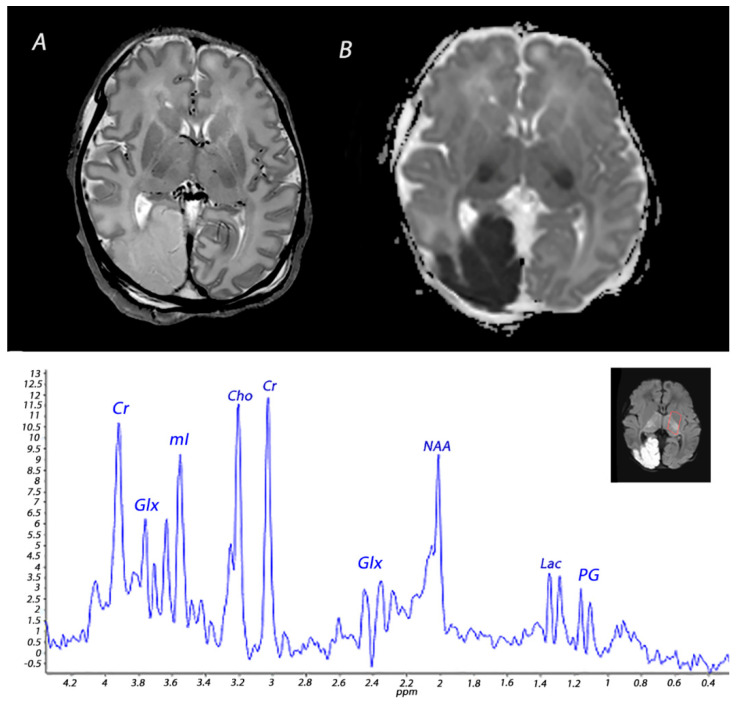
MRI performed on day 3 in an infant born with Apgar scores of 3/4/6 who received therapeutic hypothermia for severe HIE. MRI showed posterior cerebral artery stroke, presenting as increased signal intensity and loss of cortical gray and white matter differentiation on T2 weighted imaging (A) and a low signal intensity on ADC mapping (B). Injury was also noted in the BGT. ^1^H-MRS performed in the BGT shows a lactate peak, presenting as a doublet peak at 1.33 ppm.
